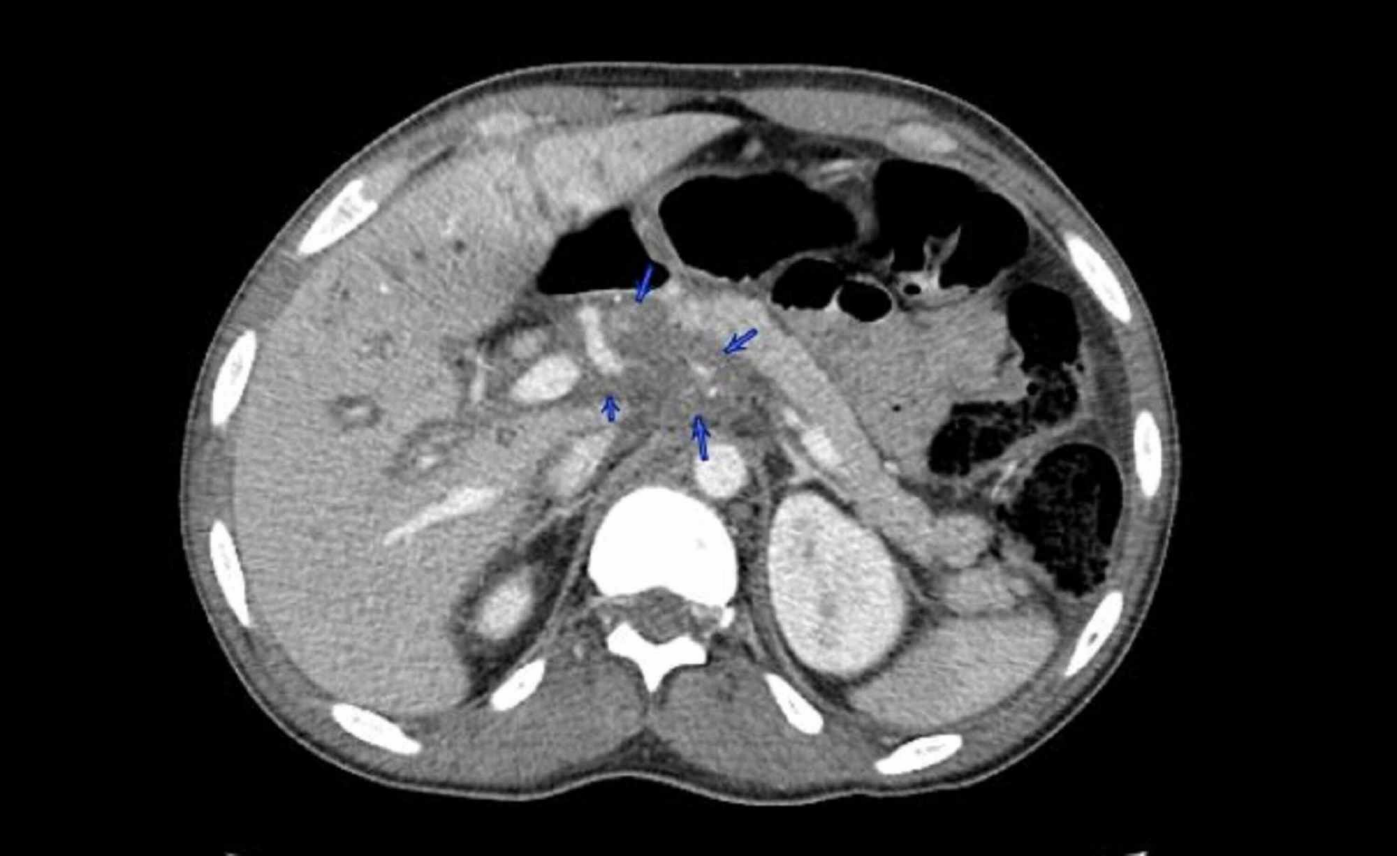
CT of the abdomen CT of the abdomen showing an infiltrative process in the portal hepatis to the celiac area (blue arrows) with obstruction of the biliary tract with intrahepatic biliary dilation. CT, computed tomography
